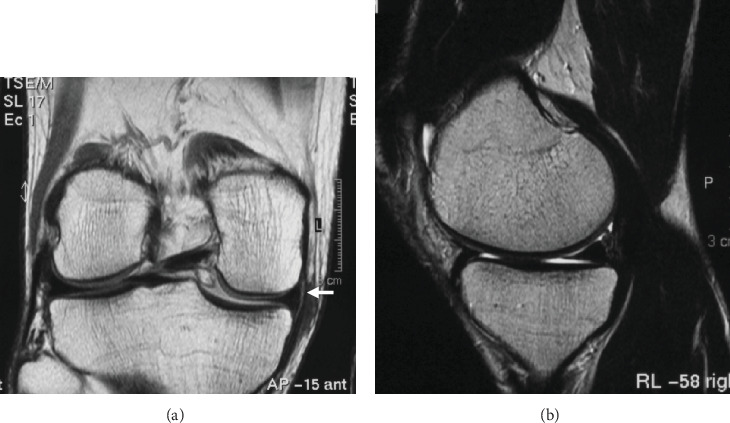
Magnetic resonance imaging findings taken at 8 years after the initial meniscal repair for the bucket handle tear of the medial meniscus. (a, b) The repaired site appears healed, and no intrameniscal degeneration is observed (white arrow).
